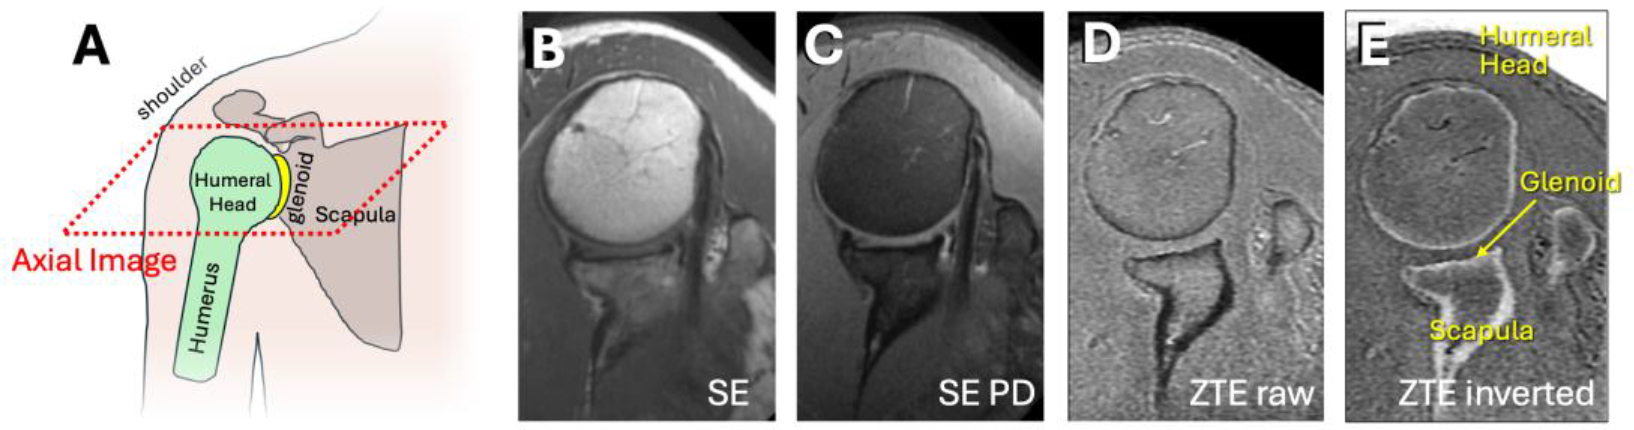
(A) Anatomy of shoulder showing major bone structures of humerus, humeral head, glenoid, scapula. Axial imaging plane is shown in dotted red box. Conventional shoulder MR images acquired using conventional (B) spin echo proton density weighted, (C) spin echo proton density weighted with fat suppression, and (D) zero echo time (ZTE) sequences. Conventional sequences do not isolate bone effectively. (E) Inverted ZTE image depicting mostly bony tissues with high signal intensity. Conventional MR images depict non-bony tissues with similar contrast as bony tissues, making them less useful for bone-only imaging.
